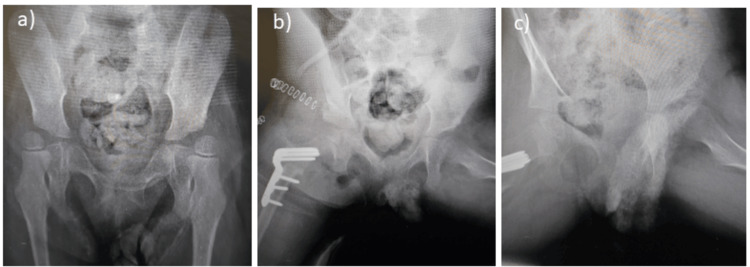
Plain x-ray of a five-year-old patient with right hip dislocation (a) Pre-operative x-ray; (b) x-ray of the same patient immediately after surgery with reduced right hip; (c) x-ray of the same patient at the 22-month follow-up showing a reduced hip joint.
